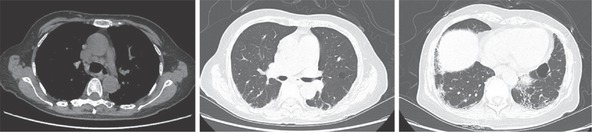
CT showing the enlargement of mediastinal lymph nodes, pulmonary bulla, and interstitial fibrosis in the base of bilateral lungs. Abbreviation: CT, computer tomography.
